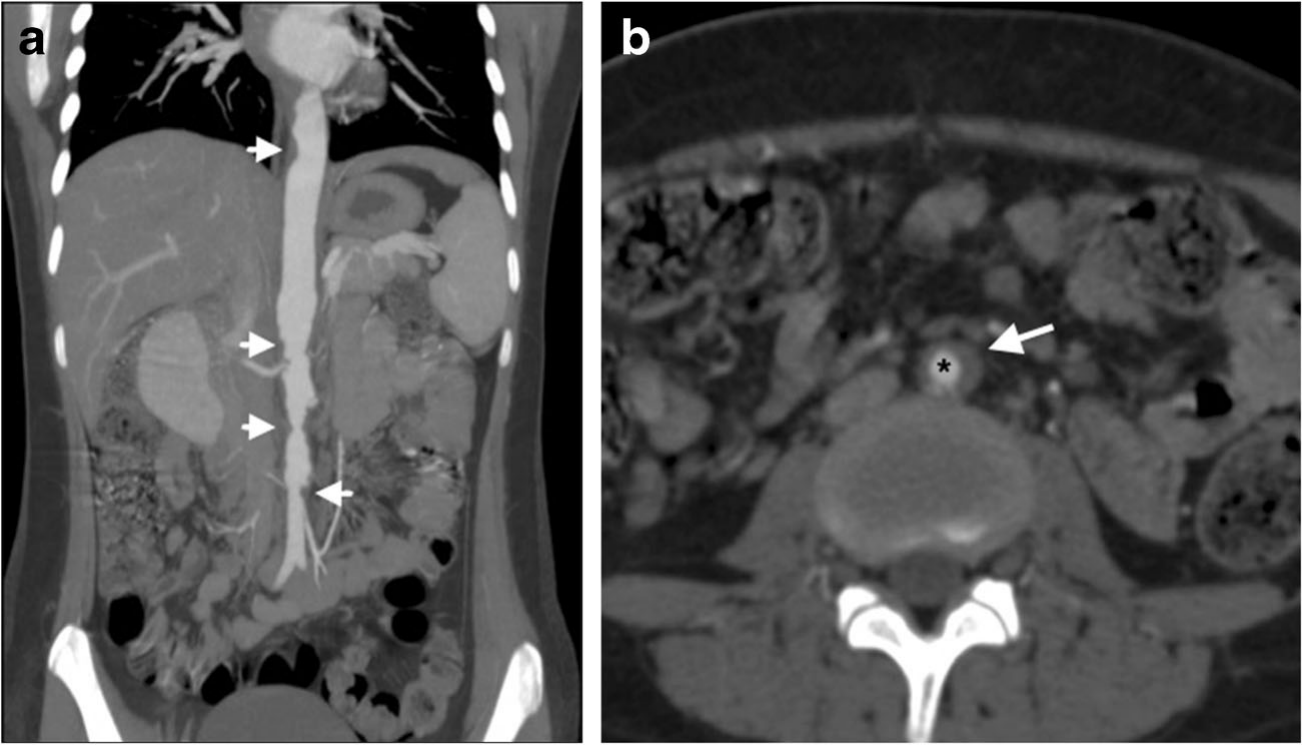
A 15-year-old girl of Indian origin presents with cough, night sweats and back pain. (a) A post-contrast CT abdominal angiogram coronal maximum intensity projection (MIP) demonstrates multifocal stenoses of the aortic hiatus and the suprarenal and infrarenal abdominal aorta (*arrowheads*). (b) An axial CT abdominal angiogram demonstrates severe luminal narrowing (*) of the infrarenal abdominal aorta, with circumferential periaortic soft tissue (*arrow*). The patient’s sputum cultures grew *M. tuberculosis*, with aortic appearance consistent with tuberculous aortitis
